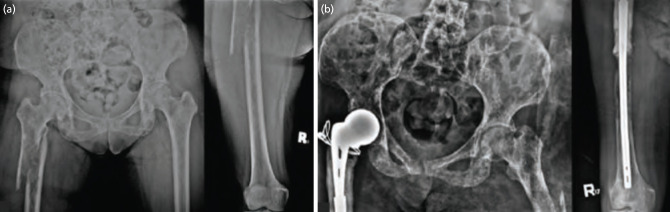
(a) Injury films of the right femur in AP view, revealing a fracture in the subtrochanteric area of the right proximal femur and a lytic lesion extending in its metadiaphyseal areas. (b) Post-operative radiographs at latest follow-up at two years, with no signs of dislocation or implant failure, but with note of progression of the lytic lesions in the pelvis and further distal to the previously applied cement, in addition to the diaphyseal fracture on the contralateral femur.
